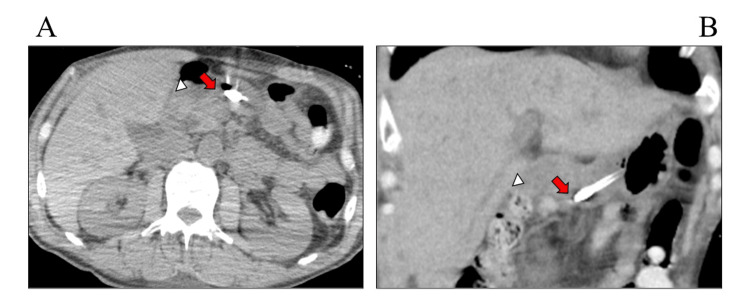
CT on day 20 revealed the tip of the enteral nutrition tube in the duodenal bulb (arrow) A: Axial scan. B: Coronal scan. Triangles show the transverse colon CT: computed tomography
